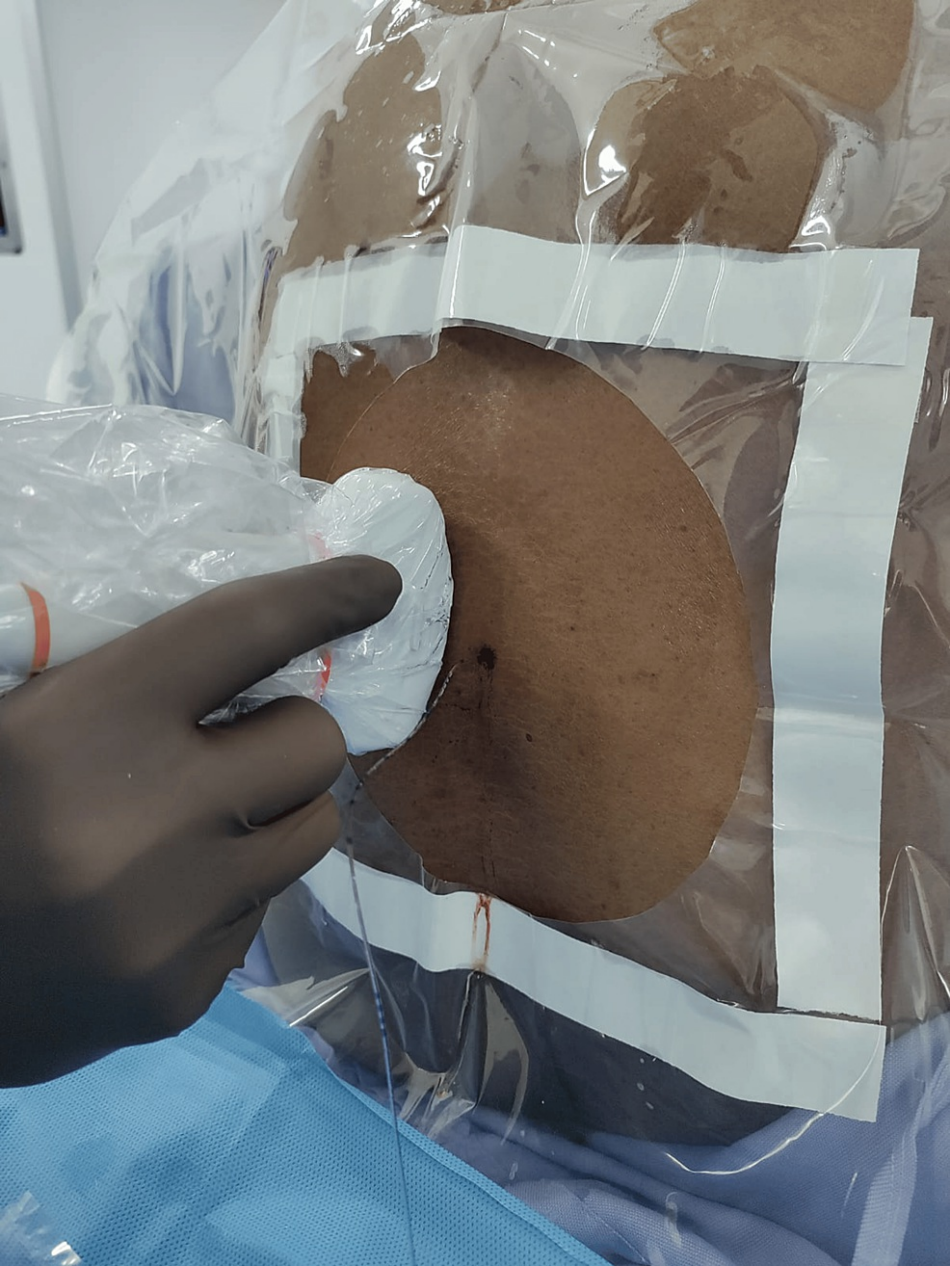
Ultrasound probe position to obtain para-sagittal oblique view of the spine
